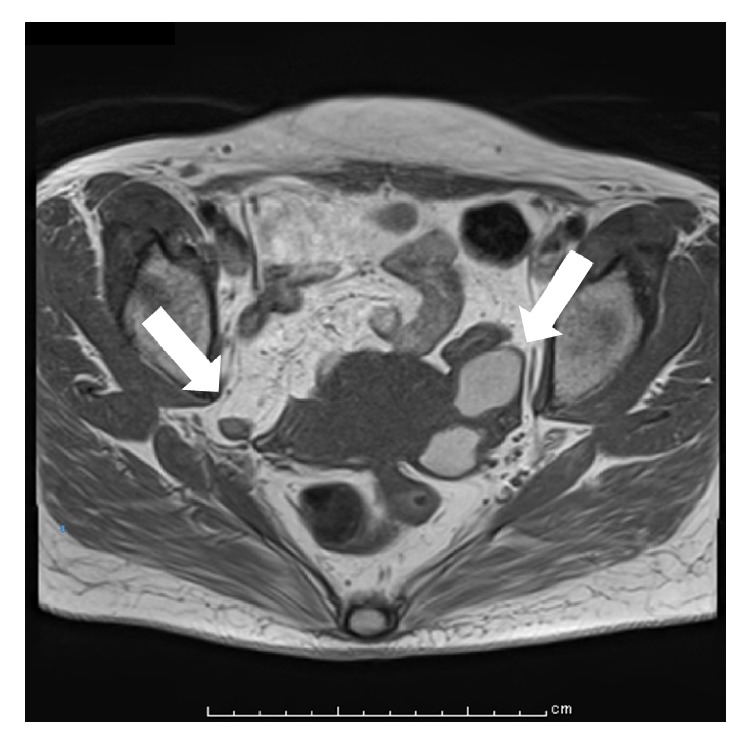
Posttreatment magnetic resonance imaging of our patient with endometriosis. Axial T1-weighted imaging after seven years of treatment. The two tumors show high-intensity signals with a diameter of 35 mm or less (arrow), and the tumor size was a partial response.
